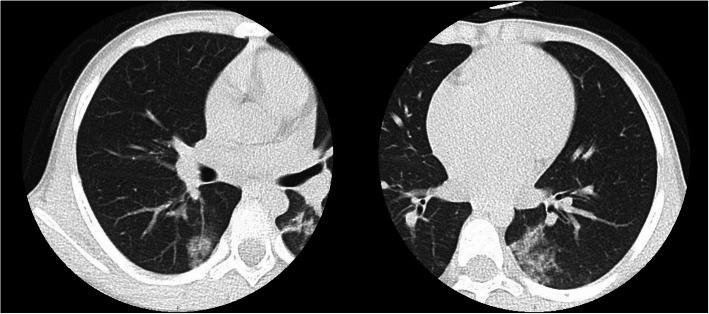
Chest high-resolution computed tomography (HRCT) scanning on admission. HRCT demonstrated patchy areas of ill-defined air-space opacity and consolidation predominantly involving the posterior basilar aspects of the lower lobes presenting with interstitial lung disease
